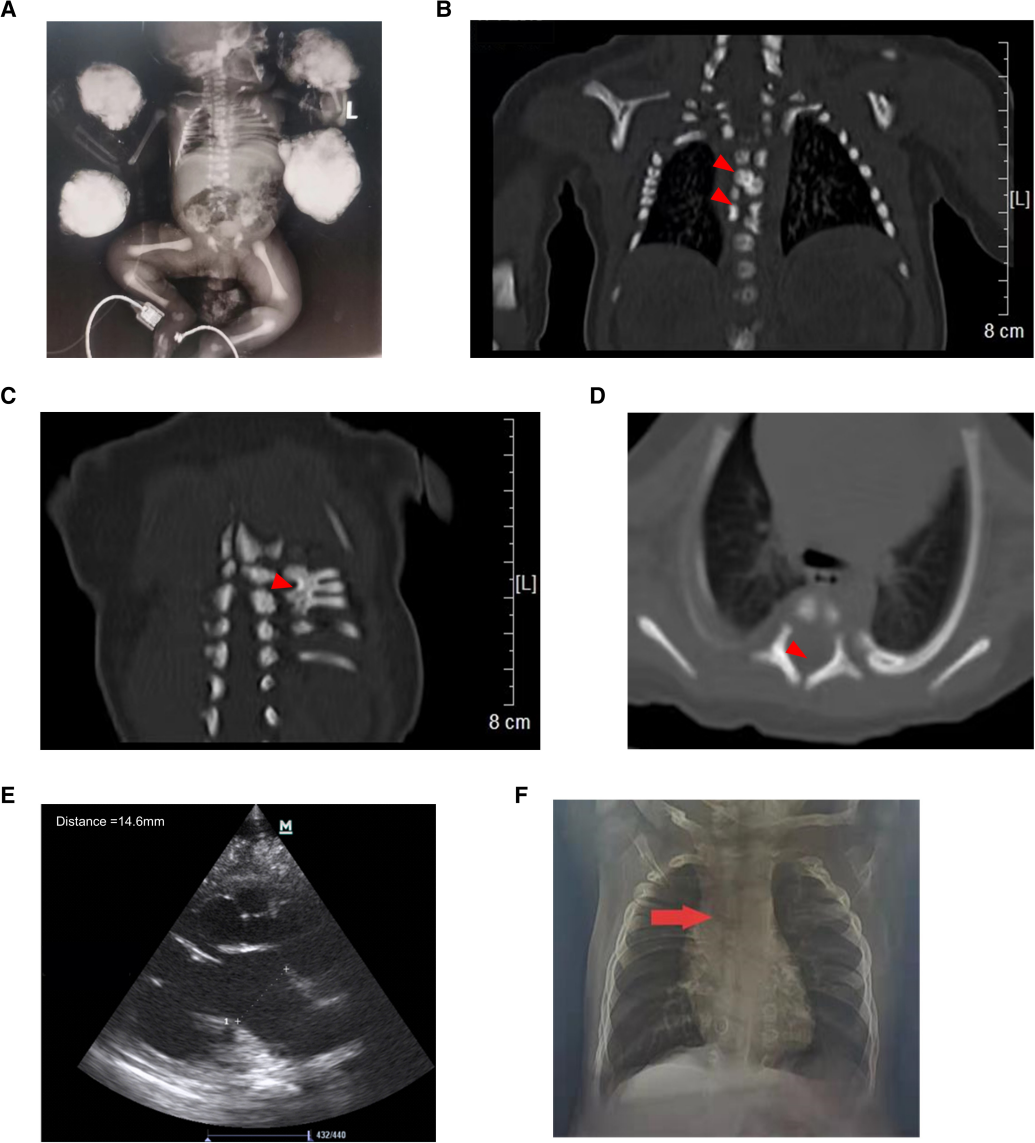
Abnormal imaging of the proband. (A) X-ray of the proband showing a short chest and distorted and fused ribs. Chest CT demonstrating hemivertebrae (B, upper arrow), butterfly vertebrae (B, lower arrow), rib fusion (C), and spinal canal enlargement (D,E) Kidney color Doppler ultrasound showing left hydronephrosis. (F) Chest x-ray showing mild scoliosis at the age of 11 months.
